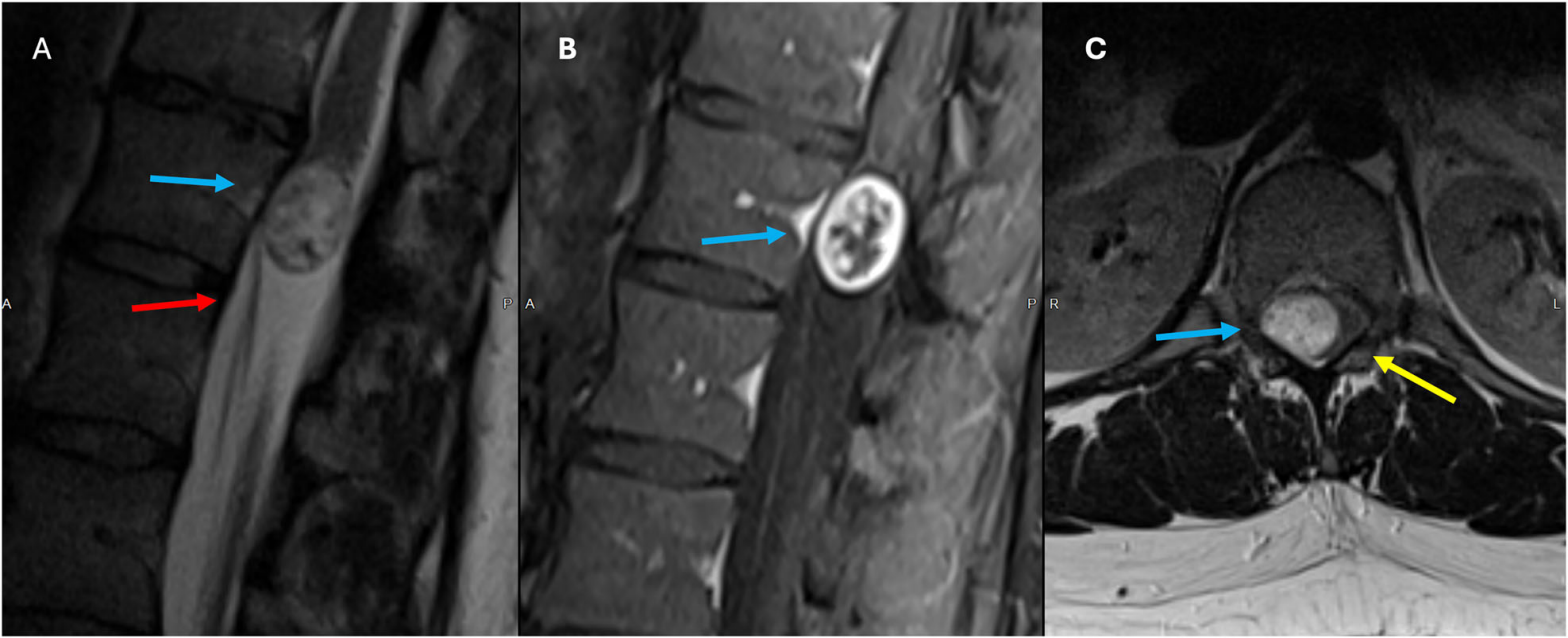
Seventy-year-old male patient with an incidentally identified mass in routine Abdominal CT (not shown). Sagittal and axial T2W (A, C) and Sagittal T1 Fatsat postcontrast (B) images showing an uniloculated cystic intradural extramedullary lesion at T11–L1 levels (blue arrows). The lesion is anteriorly displacing the cauda equina (red arrow) and compressing the conus towards the left (yellow arrow)
